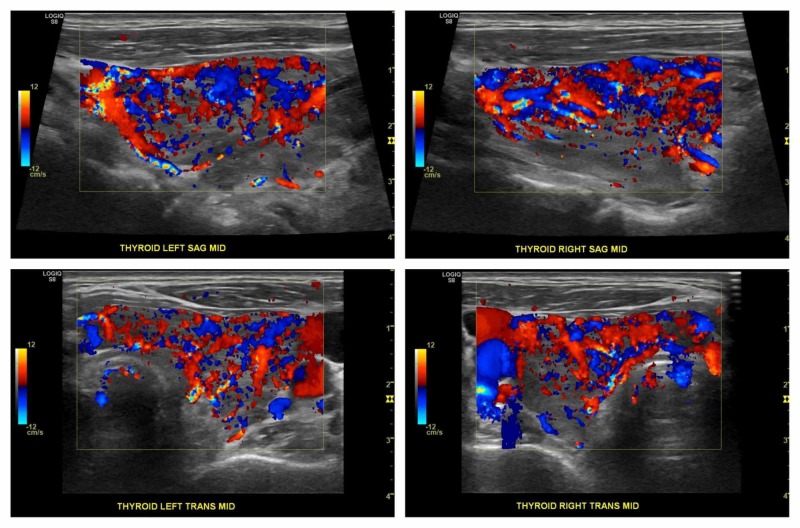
Ultrasound Doppler of the thyroid showing a hyperemic-appearing thyroid gland with a diffuse increase in blood flow in the left and right lobes of the gland Views in sagittal (SAG) (upper row) and transverse (TRANS) (lower row) planes
